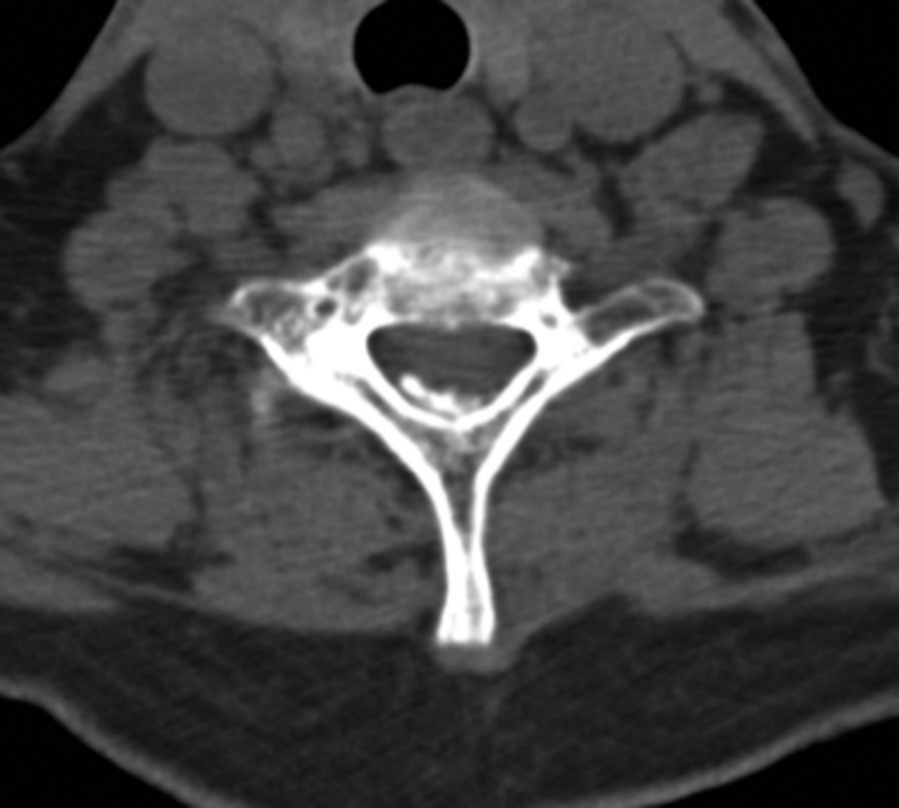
Axial CT-scan showing a hyperdense dorsal intraspinal extradural lesion at C7
